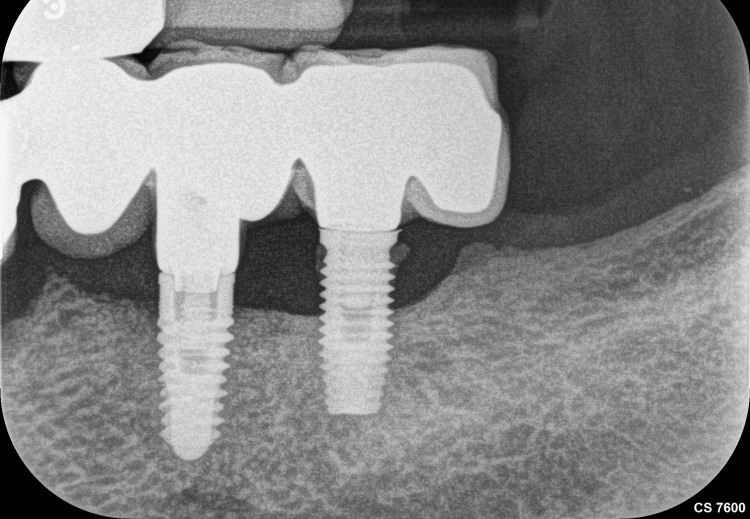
Radiographic evaluation revealed normal crestal bone height with mild bone loss involving two to three threads around each implant and the presence of calculus deposits on the distal surface of the implant. A periapical radiograph was acquired using a Carestream CS 7200 phosphor‑plate imaging system (Carestream Dental LLC, Atlanta, GA; size 2 plate). Exposure parameters: 70 kV, 7 mA, recommended exposure time 0.16 seconds for mandibular incisors/premolars as per manufacturer’s guidelines.
